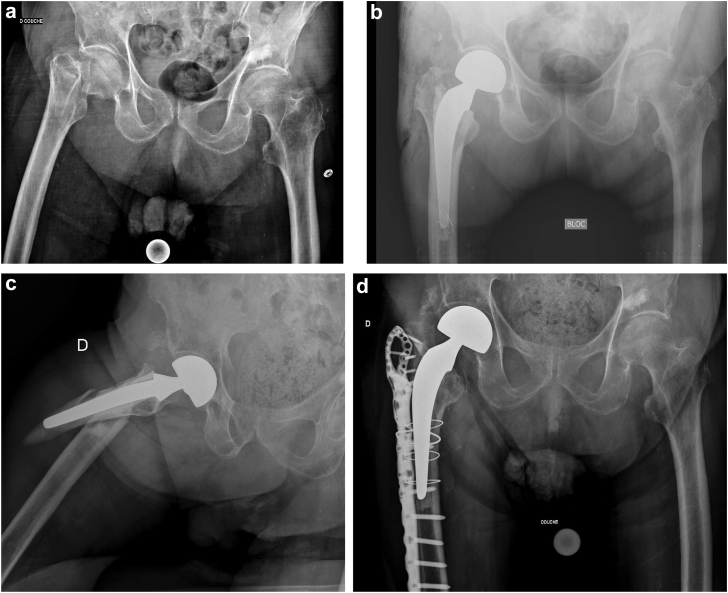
(A) Anteroposterior pelvis view of a FNF in an 82-year-old male with Dorr B type femur. (B) AP pelvis after right HA. (C) AP radiograph at 18 months postoperatively showing a PFF type B1 Vancouver following a fall. (D) AP radiograph performed in the follow-up 3 months after ORIF. ORIF, open reduction and internal fixation; FNF, femoral neck fracture.
